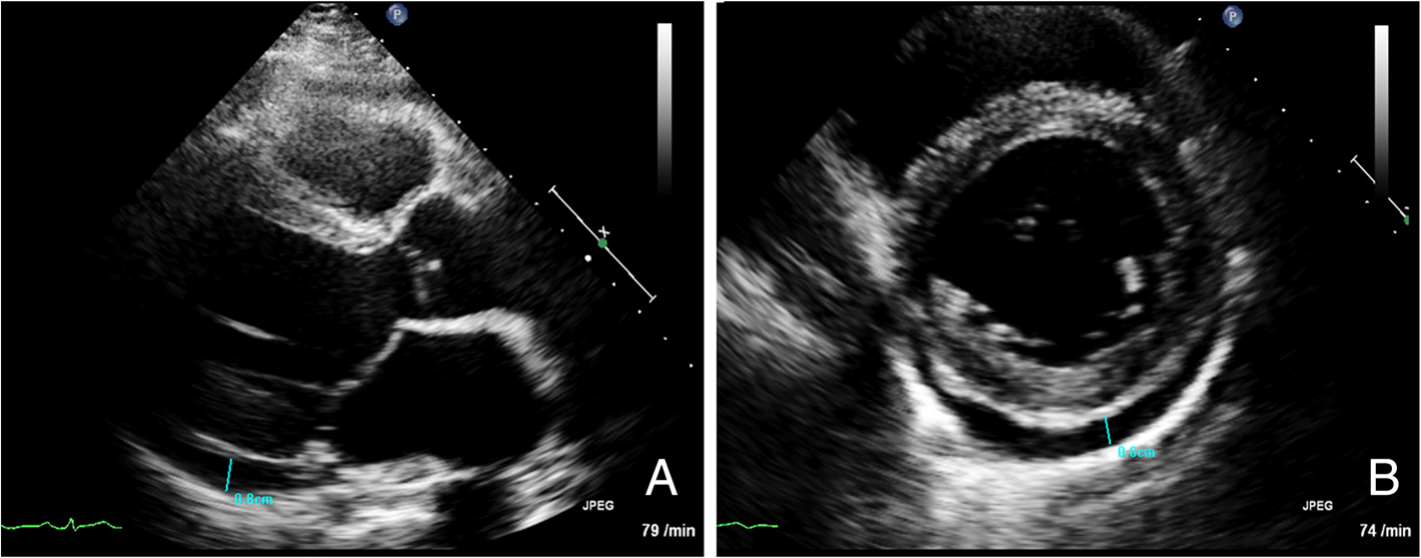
Transthoracic cardiac echocardiogramms. (A) Long axis view. (B) Short axis view. These views show a pericardial effusion of 0.8 cm at readmission.
